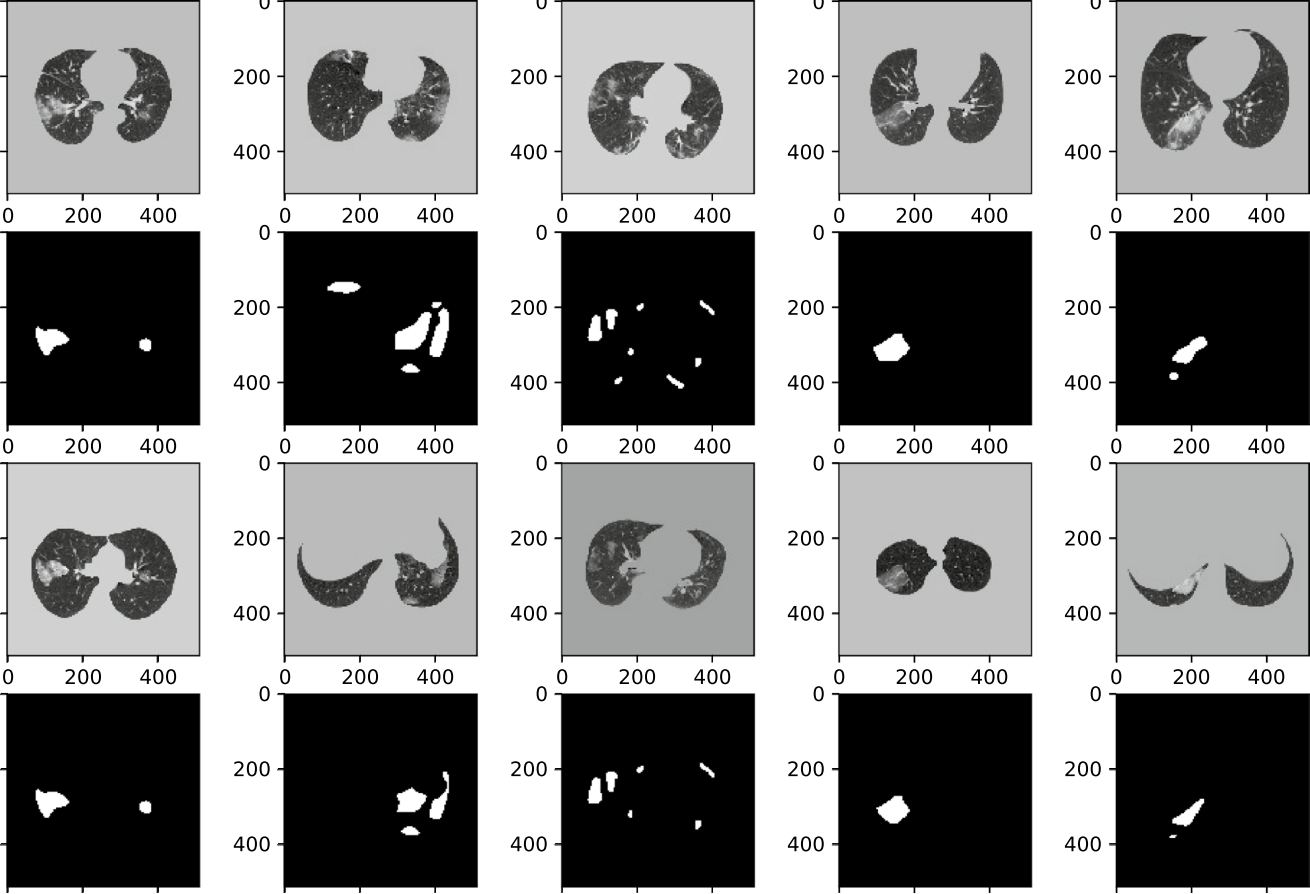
Samples of synthetic images and infection masks. First and second rows: COVID-19 infected CT images and their corresponding infection masks. Third and fourth rows: Synthetic images and the adjusted infection masks.
